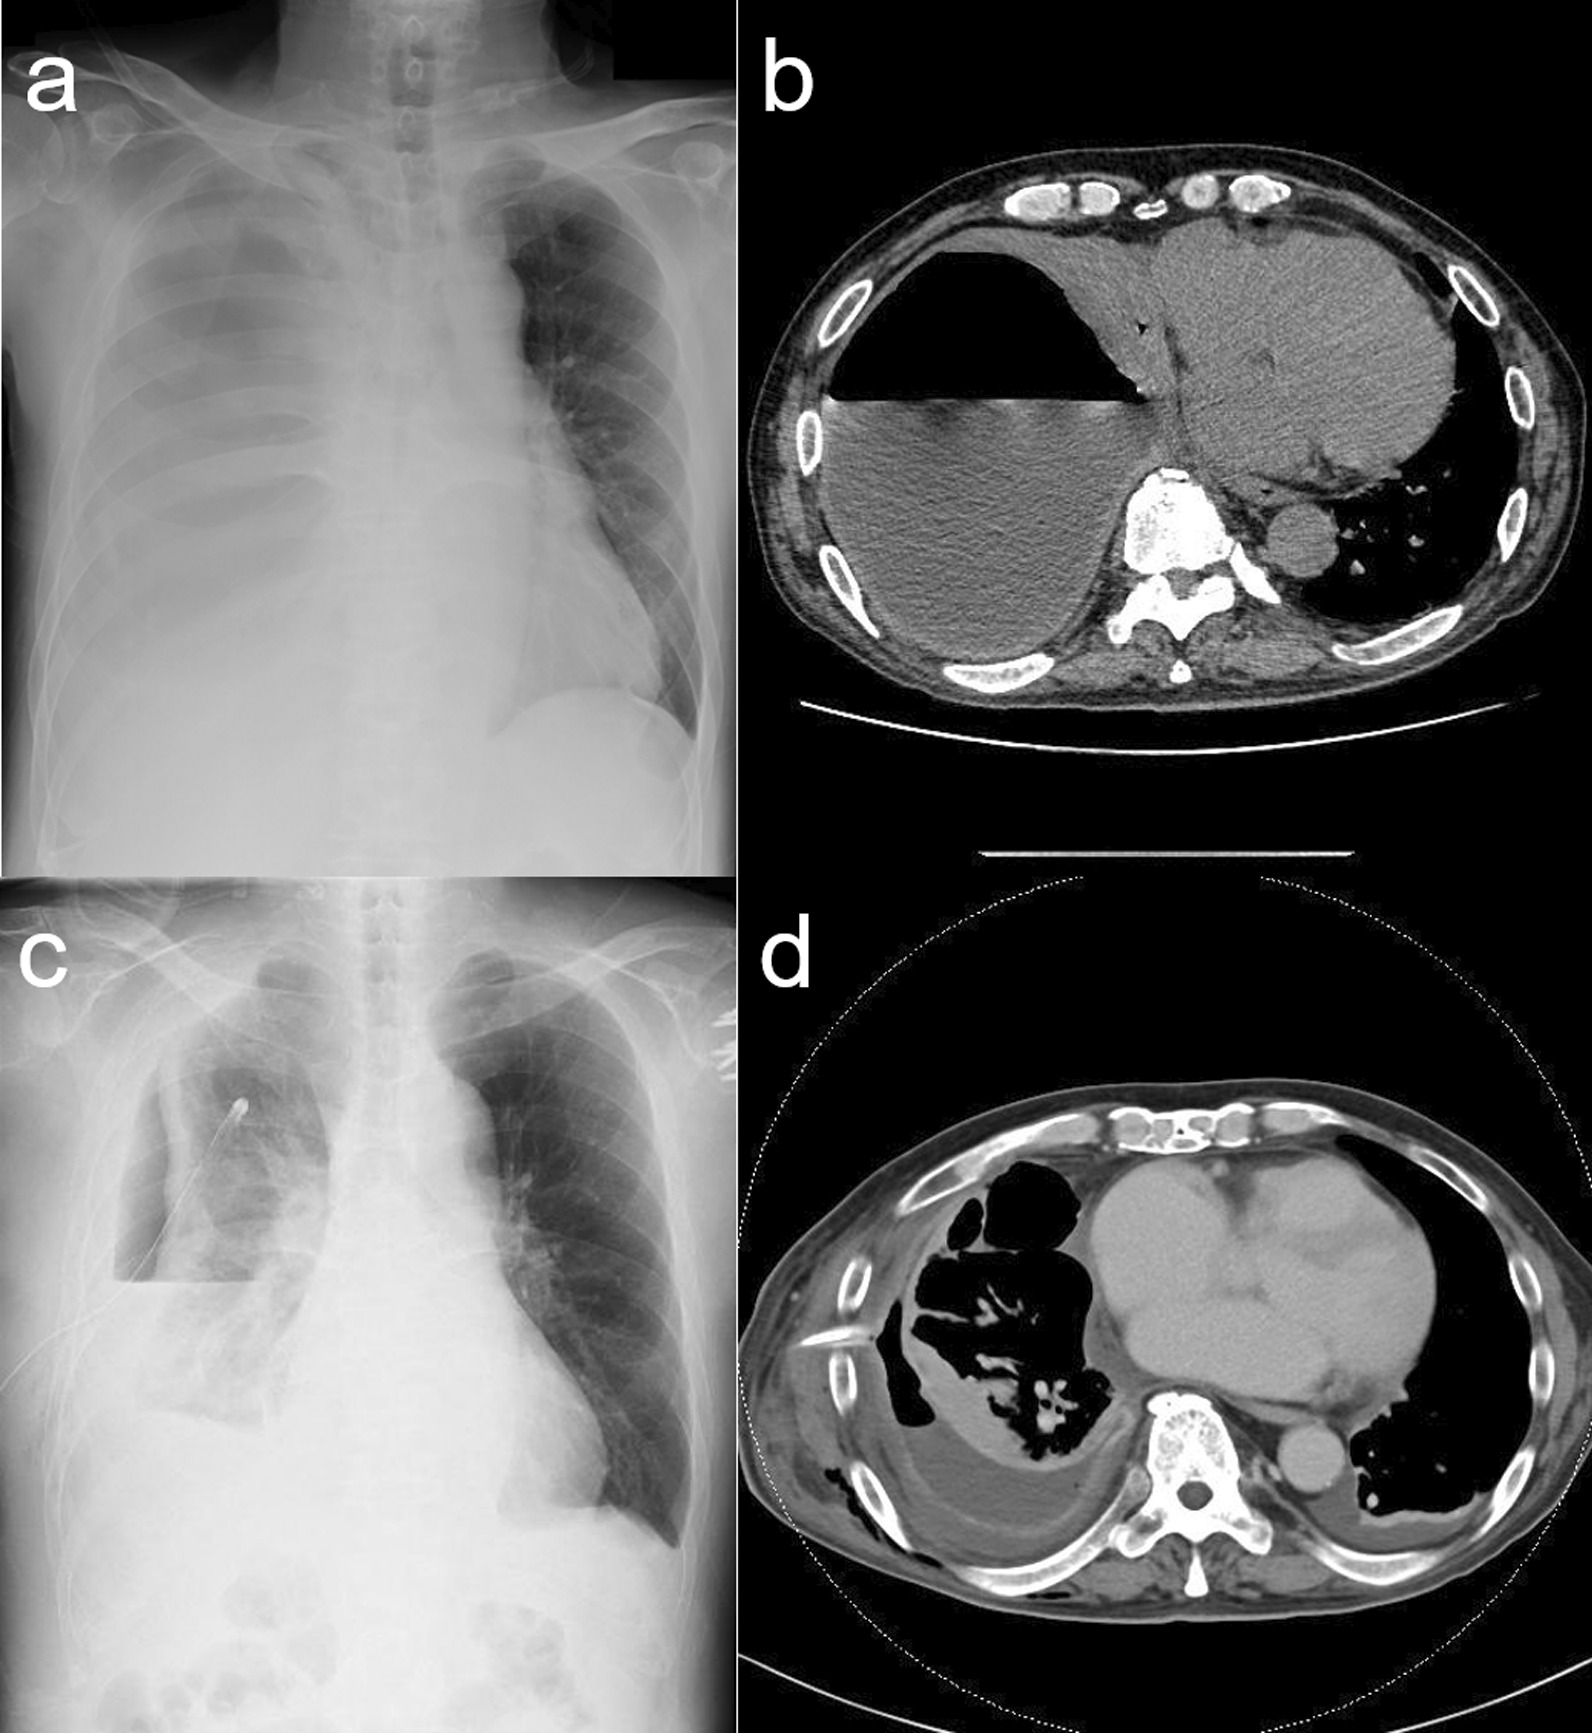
a Chest X-ray imaging and b computed tomography (CT) at the first visit. Chest X-ray imaging revealed right lung collapse and pleural effusion, and leftward deviation of the mediastinum. (Supine, anterior–posterior view) Chest CT revealed pleural effusion with air-fluid level and collapse of the right lung. c Chest X-ray and d CT imaging after drainage showed improvement in the mediastinal deviation and an expansion failure of the lung
